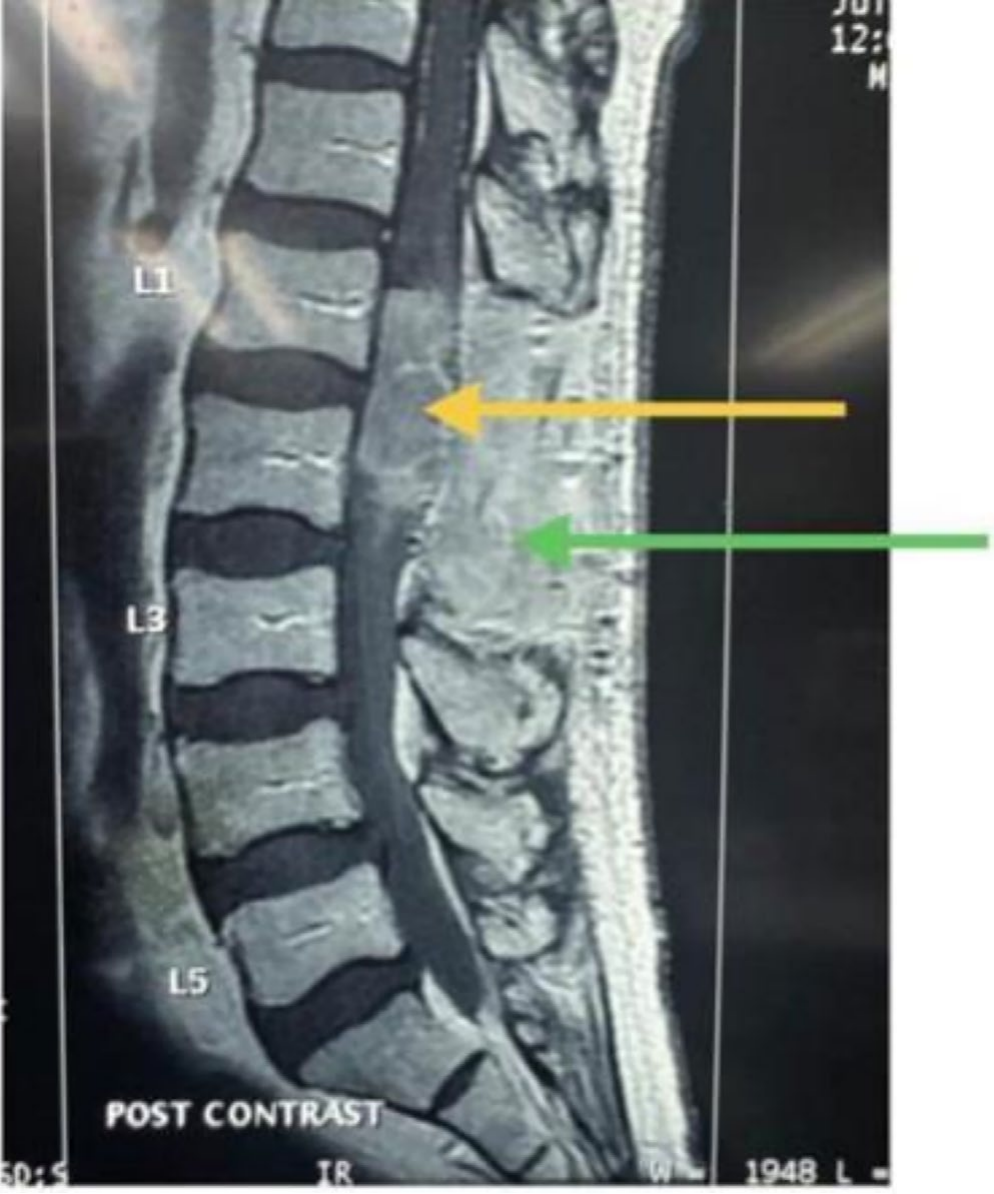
Post contrast T1 weighted image shows a 6‐month post‐laminectomy and excision MRI with contrast, showing recurrence of the tumour. The tumour extends beyond the lower endplate of L1 as seen before surgery. This shows an increase in the size of the tumour. There is an extensive contrast‐enhancing scar tissue involving the laminectomy defect. Yellow arrow shows an enhancing lesion extending from conus to cauda equina. Green arrow shows an L1–L2 lamina defect with extensive enhancing scar within the defect.
